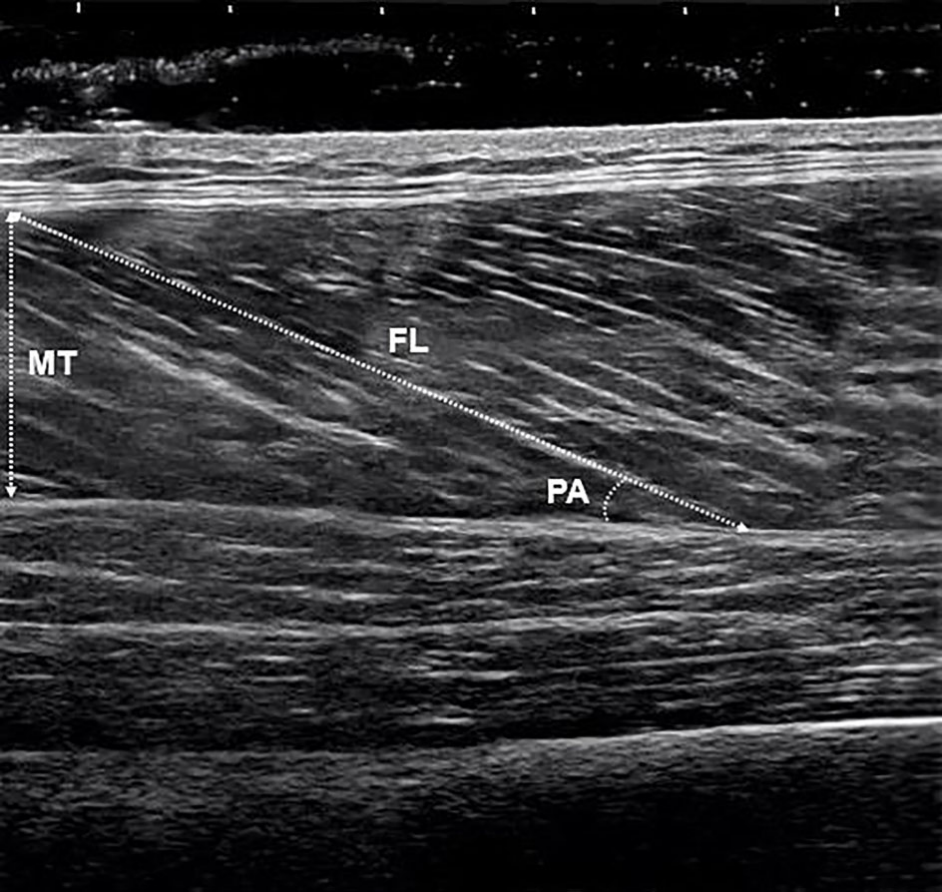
Architectural measurements of the vastus lateralis (VL) in the ultrasound image. PA: pennation angle, FL: fascicle length, MT: muscle thickness.
